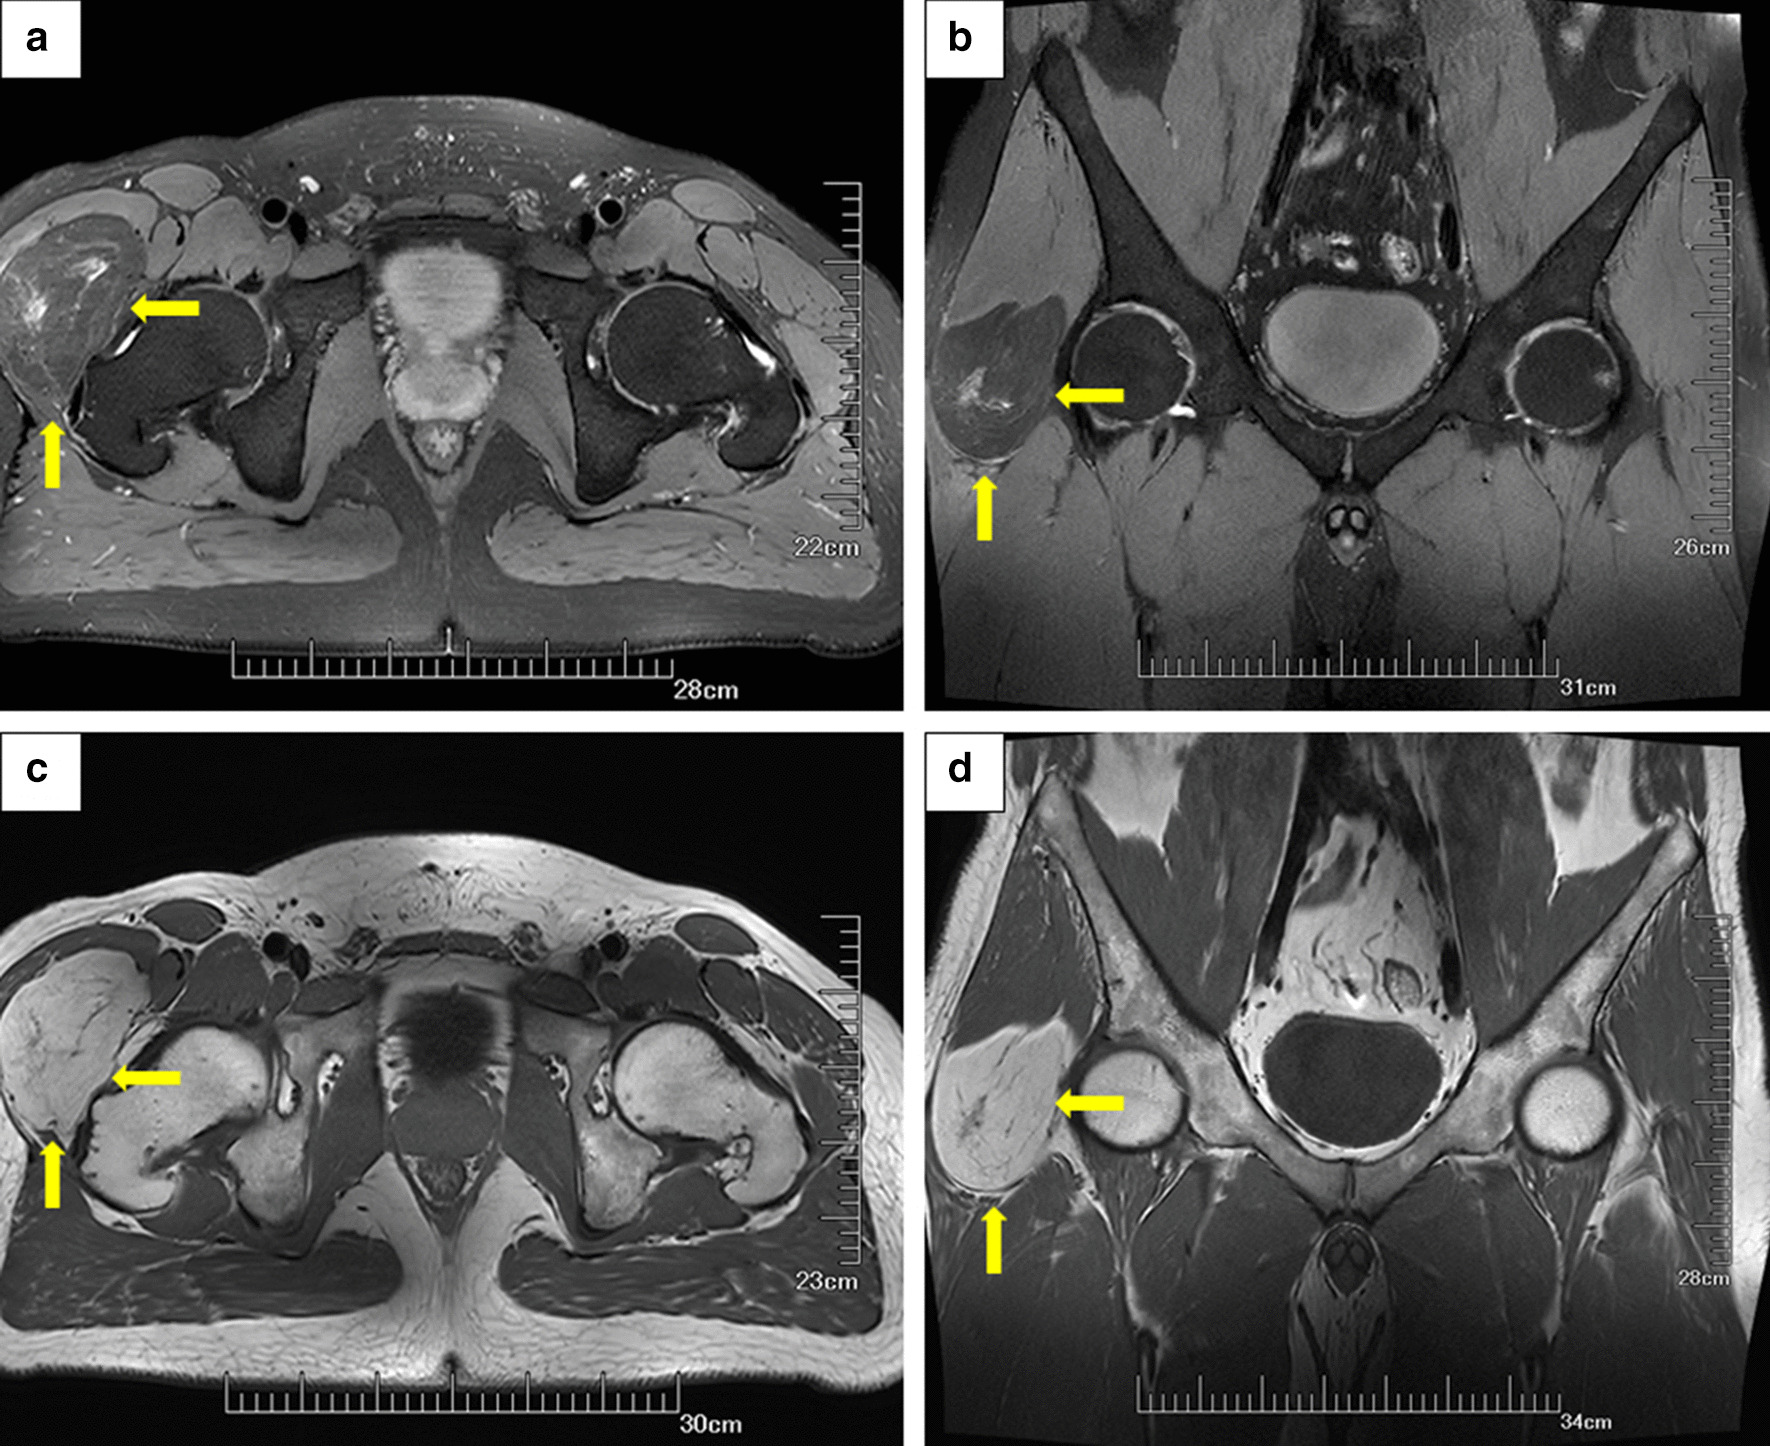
a, b Axial T1-weighted and coronal T1-weighted magnetic resonance (MR) images show lumpy shadows with abnormal signals in the lateral soft tissue space of the right hip joint with a clear boundary, a high-intensity signal shadow with a cord strip, and a cloud-like low-intensity signal shadow. c, d Axial and coronal fat-suppressed T2-weighted MR images showing a low-density signal shadow with a cord strip and a cloud-like high-intensity signal shadow. The adjacent muscles are compressed and deformed by the tumor (yellow arrow)
